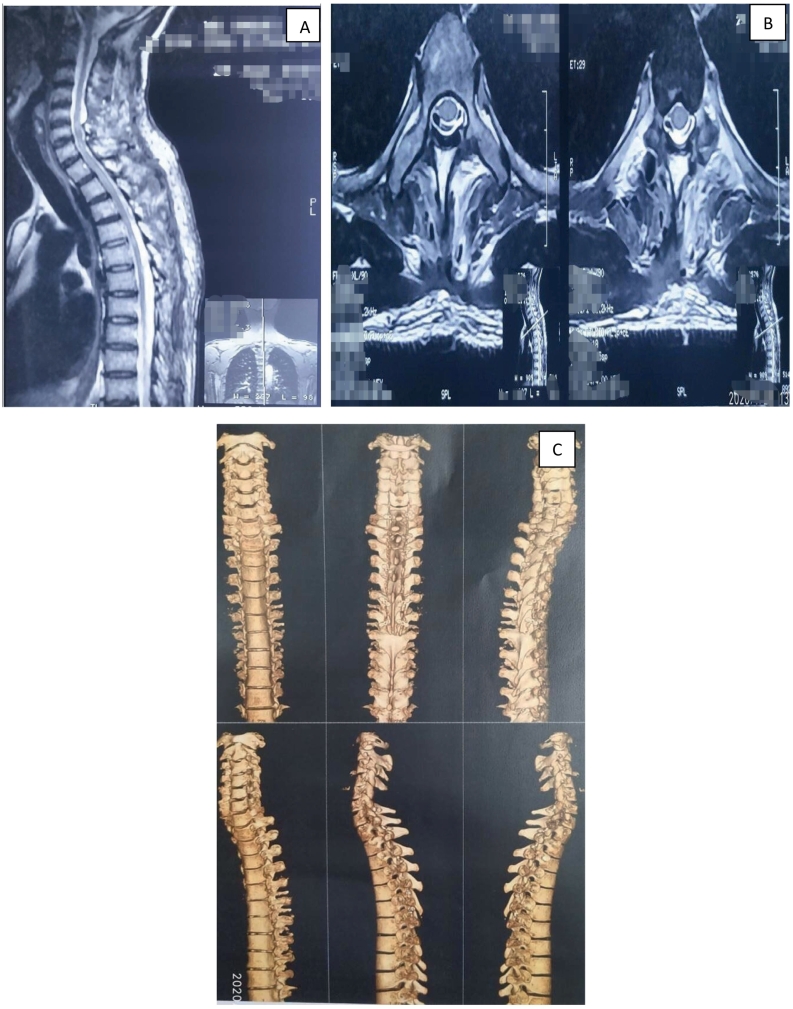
A- Postoperative T2 saggital MRI scan; B- Postoperative T2 axial MRI scan; C- 3D CT scan showing laminoplasty.
